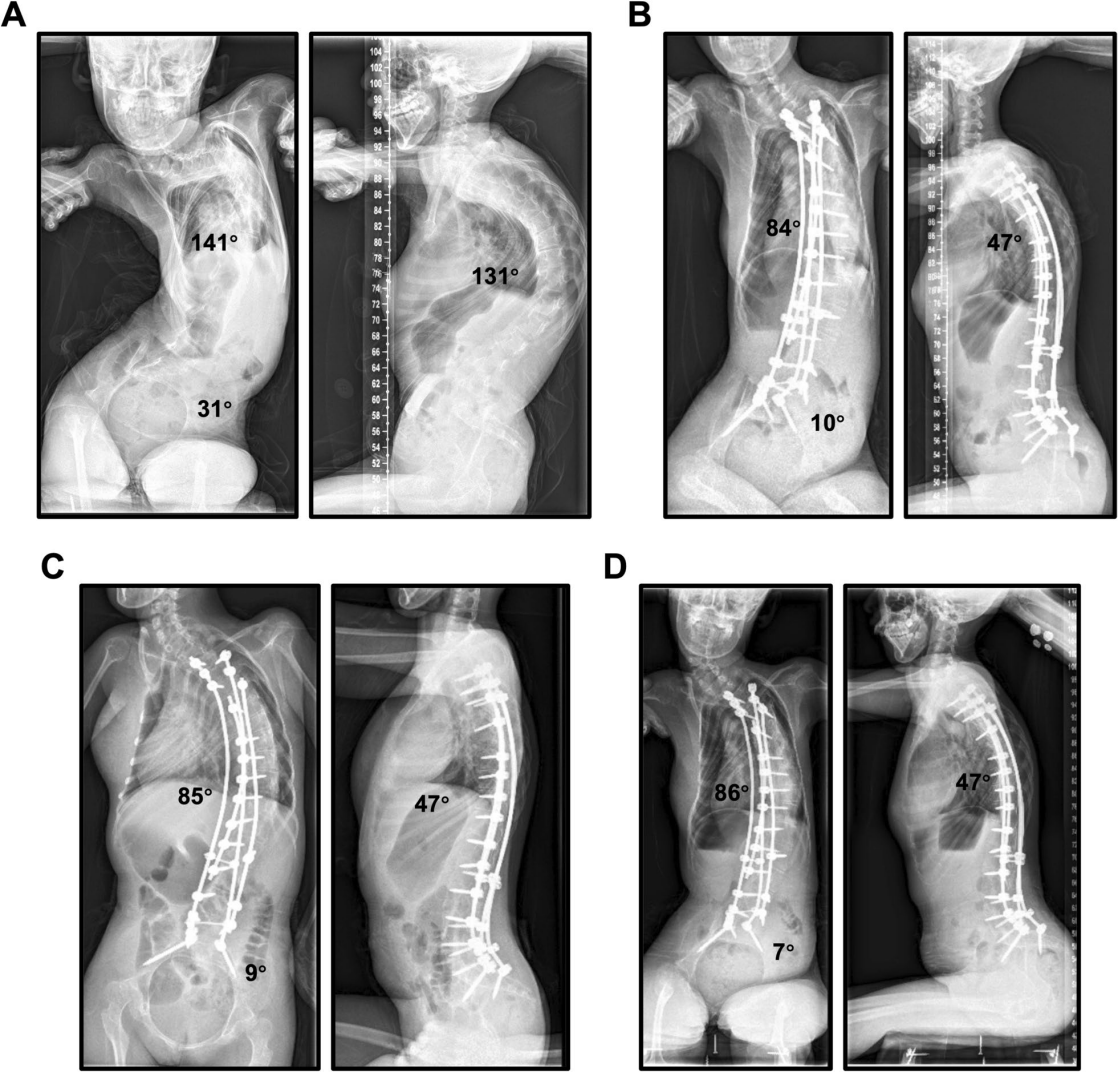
A 11-year-old female diagnosed with SMA underwent posterior spinal fusion and pelvic fixation by using traditional iliac screws (left side: 7.0 * 65 mm; right side: 7.0 * 40 mm). (A) Preoperative posteroanterior and lateral radiographs of the sitting whole-spine showing severe scoliosis with associated increased kyphosis and pelvic obliquity. (B) This image shows that spinal deformity and pelvic obliquity were corrected at 6-mo postoperatively. Images (C) and (D) show that the corrected spinal deformity and pelvic obliquity were maintained at 18-months and 64-months postoperatively, respectively
